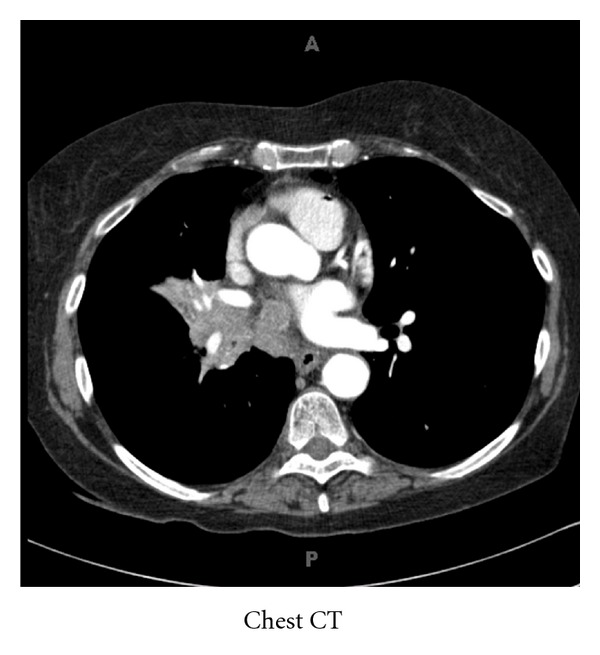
Chest CT: 6 cm right infrahilar mass with involvement of the subcarinal and paraesophageal nodes.
